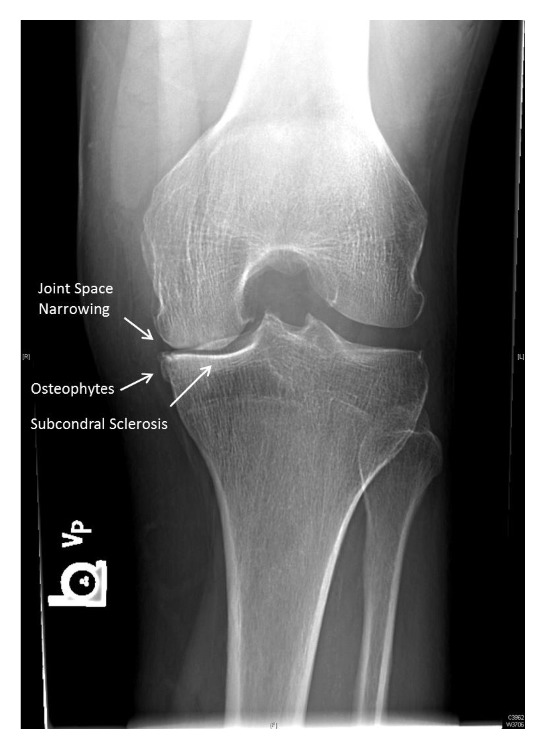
Examples of JSN, osteophytes, and subchondral sclerosis on a tunnel view radiograph.
